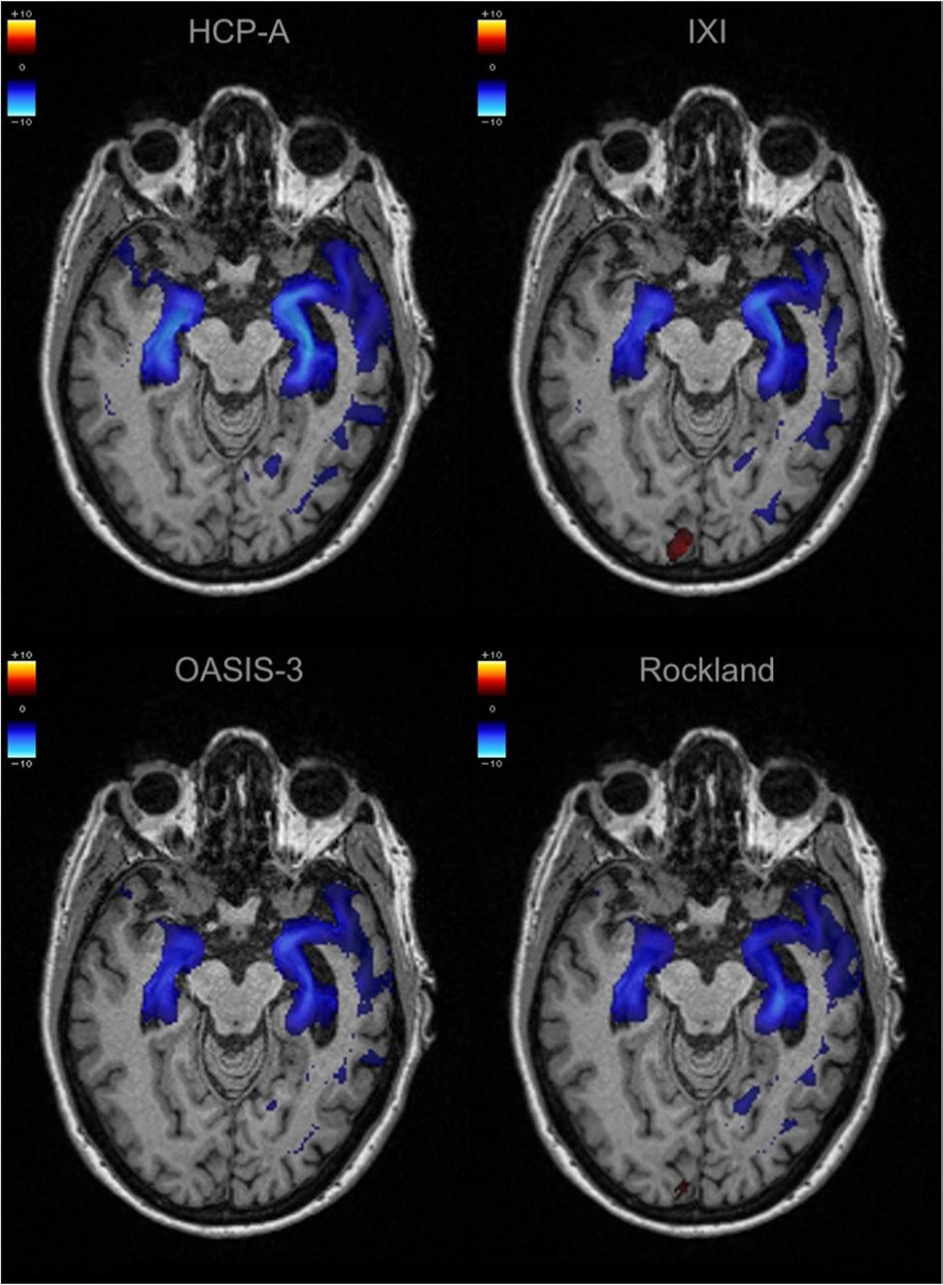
Example of the color-coded z-score maps (= atrophy maps) calculated using veganbagel for a female patient with Alzheimer’s disease (AD), aged 67 years, derived using the four different normal cohorts. The color-coded *z*-score maps are overlaid onto the original 3D T1-weighted MRI acquisitions, shown in the axial plane
